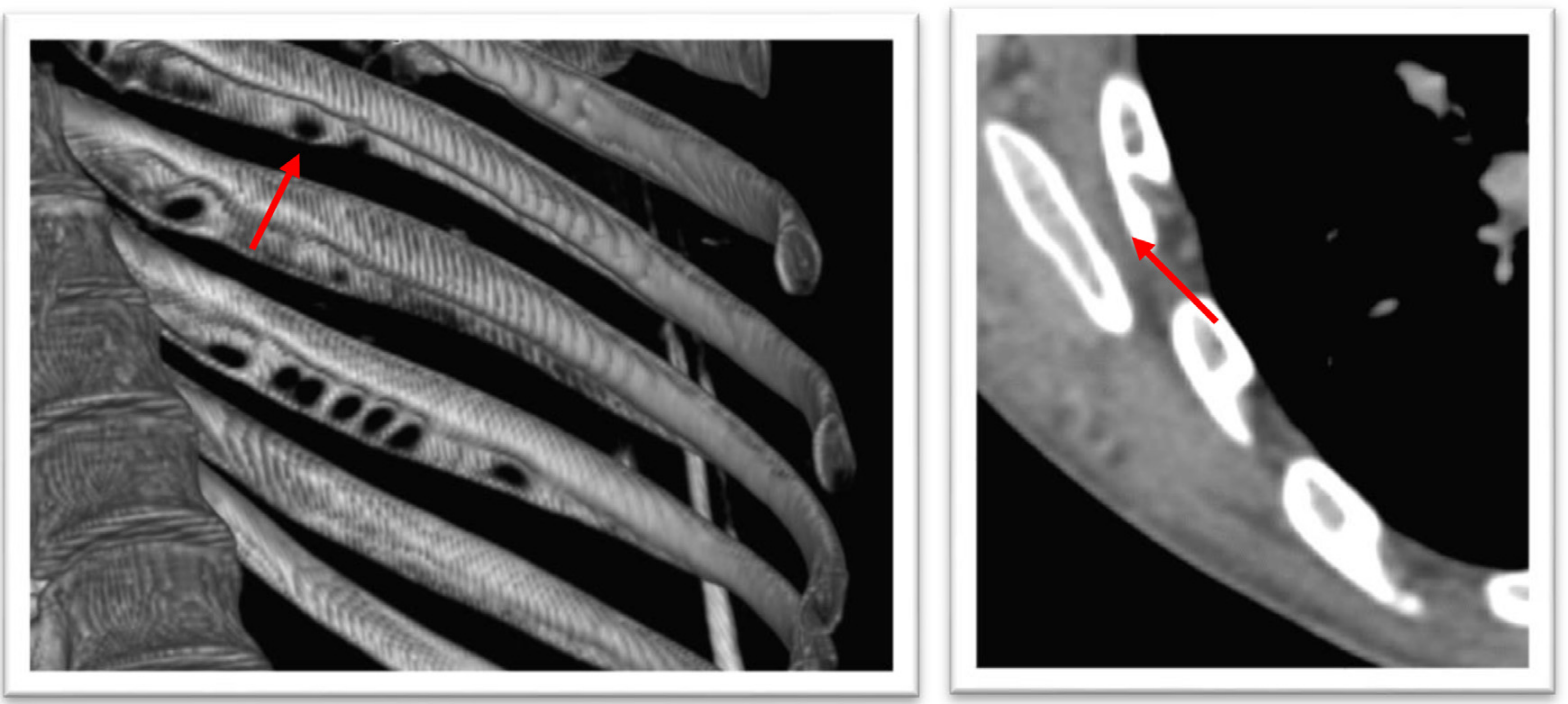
CT-scan 3D reconstruction: Inferior rib notching (red arrow): Roesler sign, secondary to dilated intercostal collateral vessels which form as a way to bypass the coarctation and supply the descending aorta. • The dilated and tortuous vessels erode the inferior margins of the ribs, resulting in notching.
